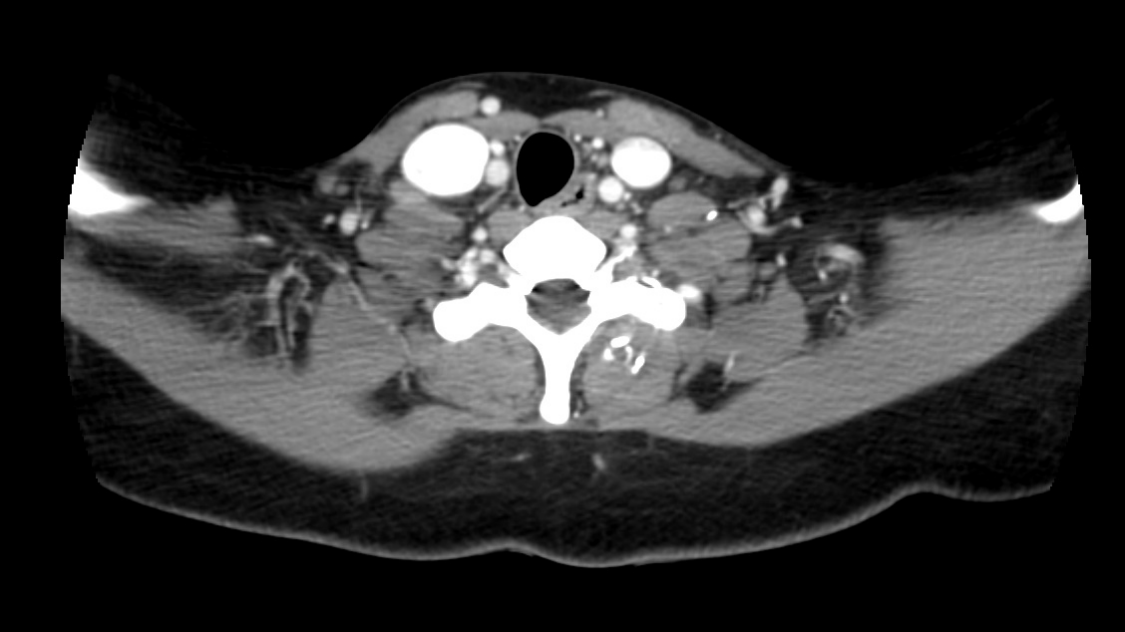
Computed tomography scan of the neck one year after initiation of anti-tuberculosis therapy
